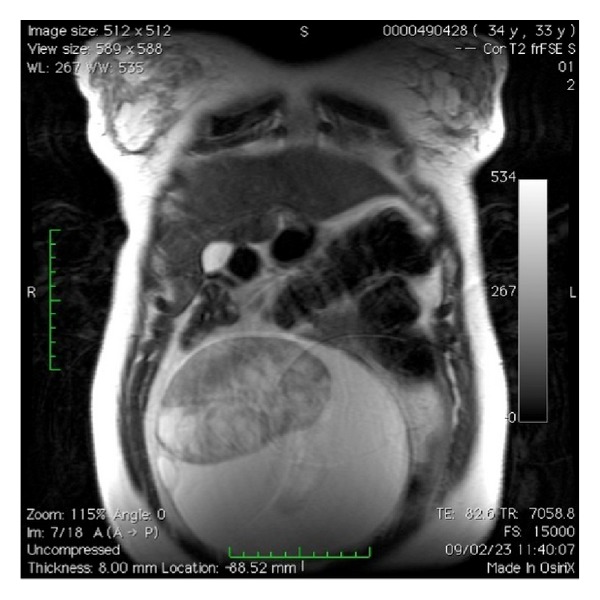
MRI showing the solid component of the adnexal mass.
